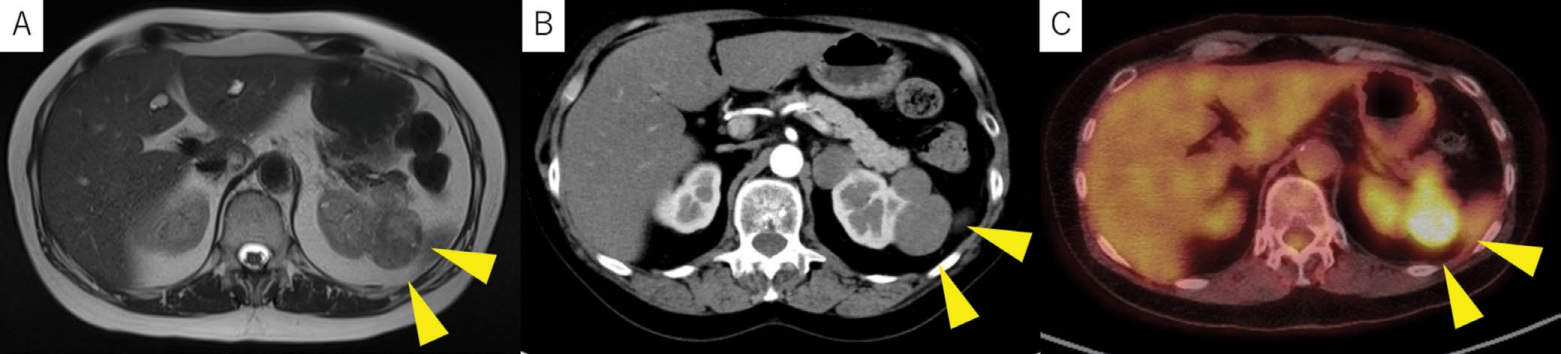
Imaging of recurrent tumor. (A) Magnetic resonance imaging showing a 36‐mm solid mass in the left renal bed with heterogeneous signal intensity on T2‐weighted imaging. (B) Contrast‐enhanced CT demonstrating heterogeneous enhancement of the lesion. (C) PET‐CT showing moderate FDG uptake in the recurrent mass.
